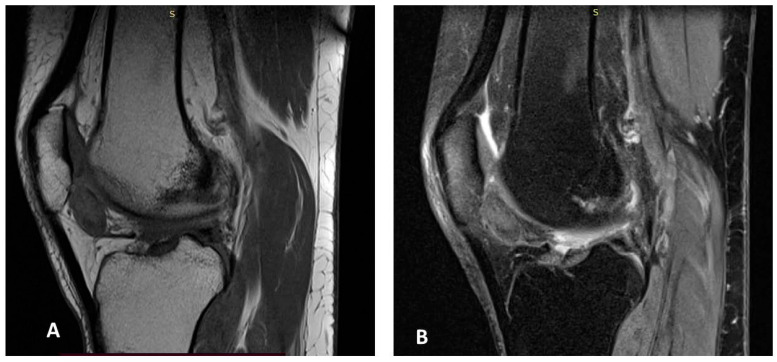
MR images of the right knee (A,B). Sagittal T1-weighted (A) and gradient-echo (B) MR images of the right knee showing an intraarticular soft-tissue mass with foci of low signal intensity in the anterior compartment, consistent with hemosiderin deposition.
